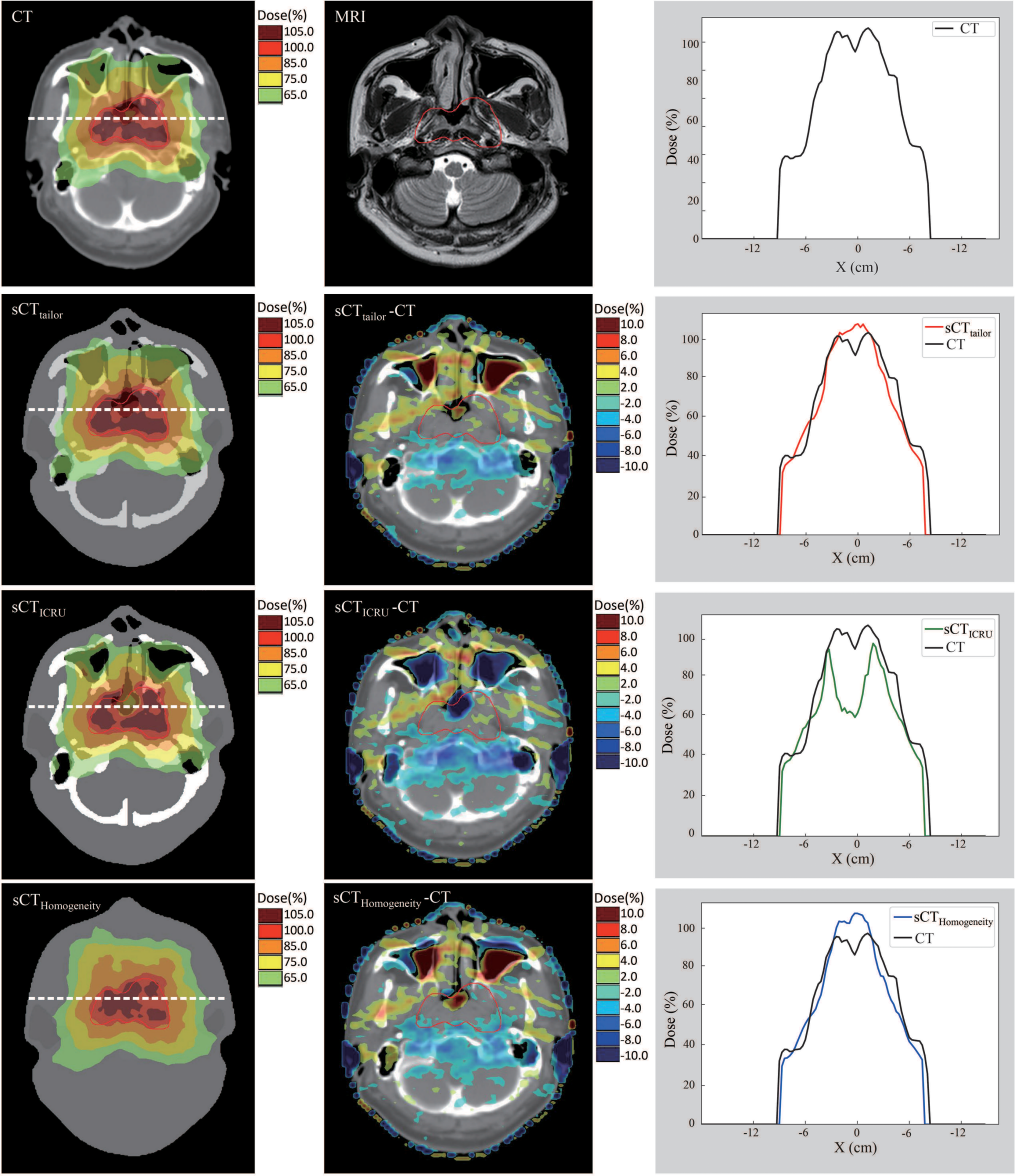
Dose comparison of plans on sCT_tailor_, sCT_ICRU_, and sCT_Homogeneity_ with respect to the original CT for dose difference maps and line profile for one NPC case.
